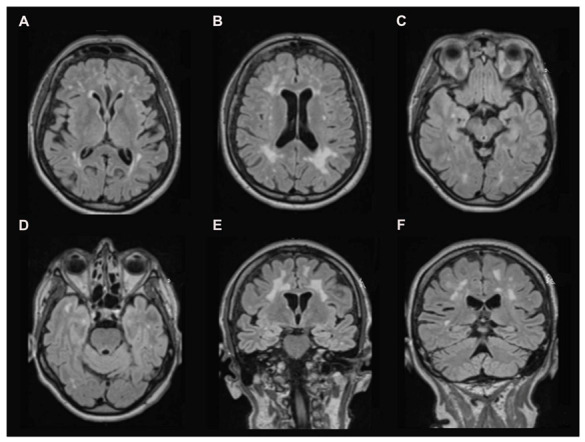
(A–C) Axial T2-weighted magnetic resonance images show a high signal intensity in patchy, confluent periventricular and subcortical areas of the white matter with a clear predominance in supratentorial areas. The basal ganglia and brainstem did not show any abnormalities. (D) Axial T2-weighted magnetic resonance images and (E) coronal T2-weighted magnetic resonance images show a slight temporal lobe involvement. (F) Coronal T2-weighted magnetic resonance images show no cerebellar abnormalities.
